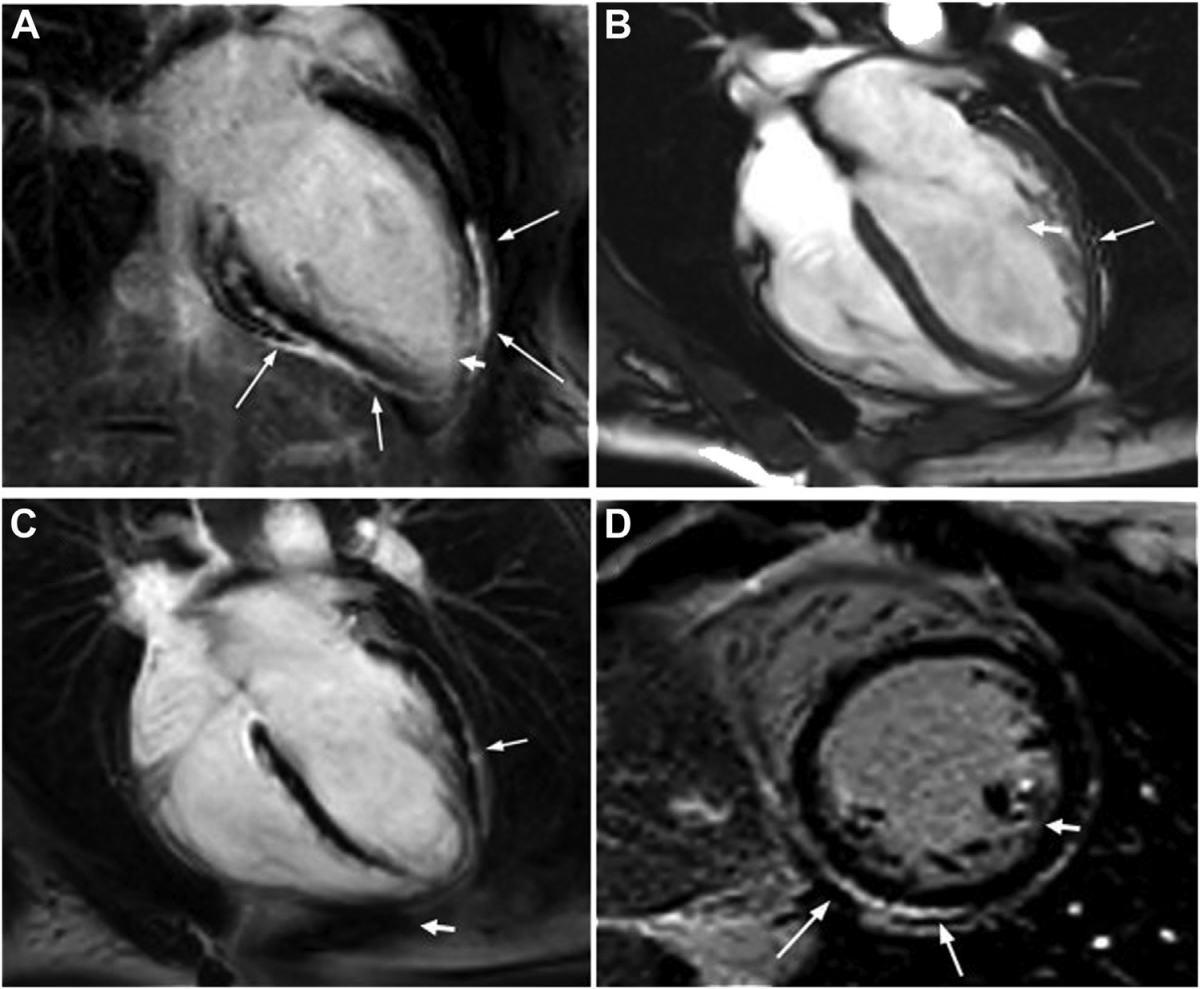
Magnetic resonance imaging (MRI) findings. A, B: Four-chamber long axis. C: Two-chamber view. D: Short-axis view. MRI shows normal left and right ventricular sizes. Panels A and B show fatty replacement within the left ventricular myocardium (white arrows). Panels C and D are left ventricular late gadolinium enhancement pattern, which is typical for arrhythmogenic cardiomyopathy (white arrows).
